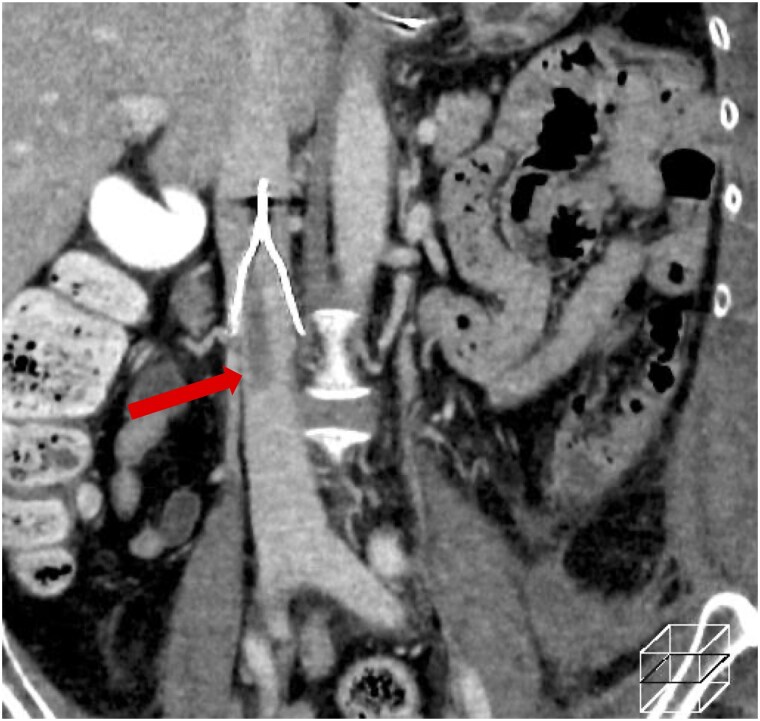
Contrast-enhanced computed tomography confirms capture of the thrombus within the inferior vena cava filter (arrow).
